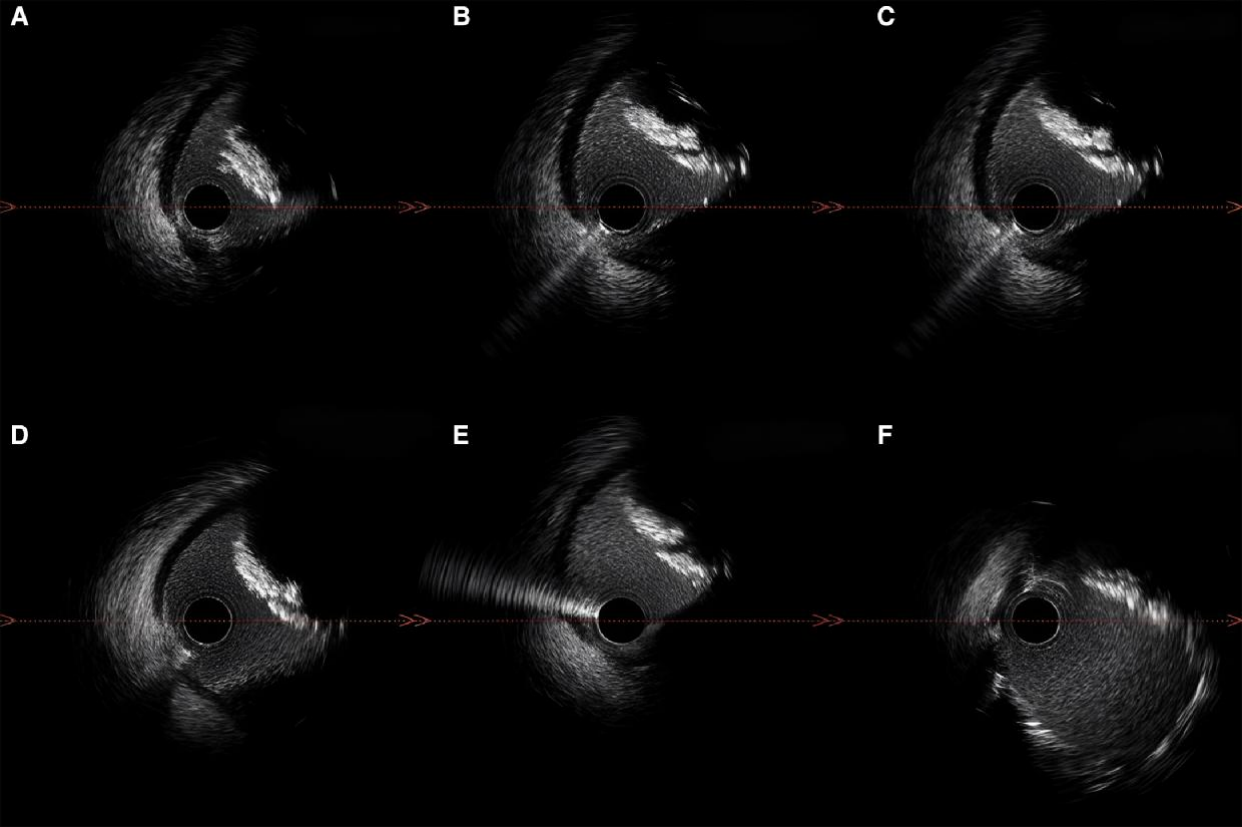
Intravascular ultrasound images of the right coronary artery showing eccentric calcified nodule without any cracks post-rotablation *(A)*, then cracked calcium nodule at multiple levels following intravascular lithotripsy *(B–E)*, and final imaging post-stenting showing good stent expansion and proper apposition *(F)*.
